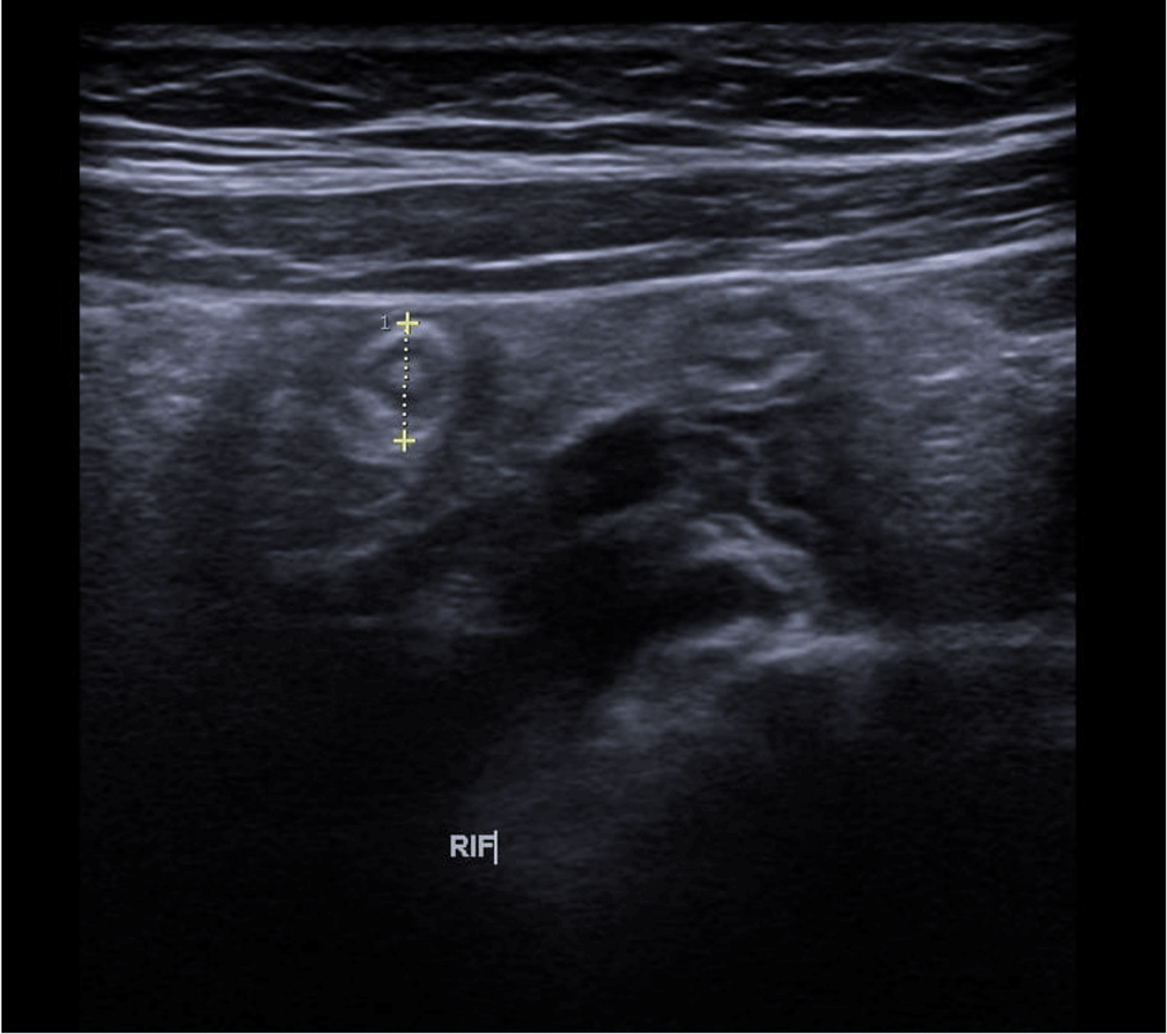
Abdominal ultrasonography demonstrating a non-compressible, dilated appendix (6 mm diameter) located in the RIF, consistent with early acute appendicitis RIF: right iliac fossa
